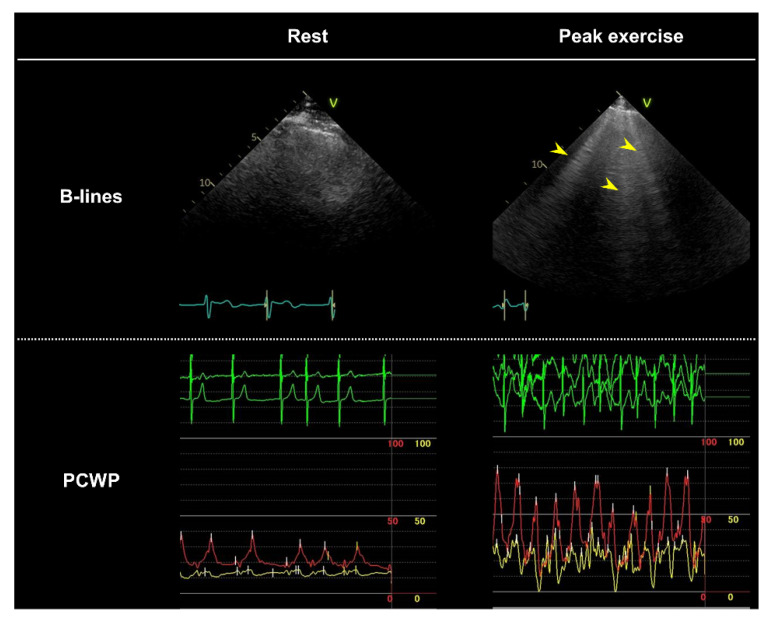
Exercise-induced ultrasound B-lines with simultaneously measured pulmonary capillary wedge pressure (PCWP) in a patient with heart failure and preserved ejection fraction. The patient demonstrated mildly elevated PCWP (19 mmHg, red line) without ultrasound B-lines at rest. During peak exercise (40 watts), the PCWP increased to 33 mmHg with marked V waves (71 mmHg), and multiple B-lines developed (yellow arrowheads).
